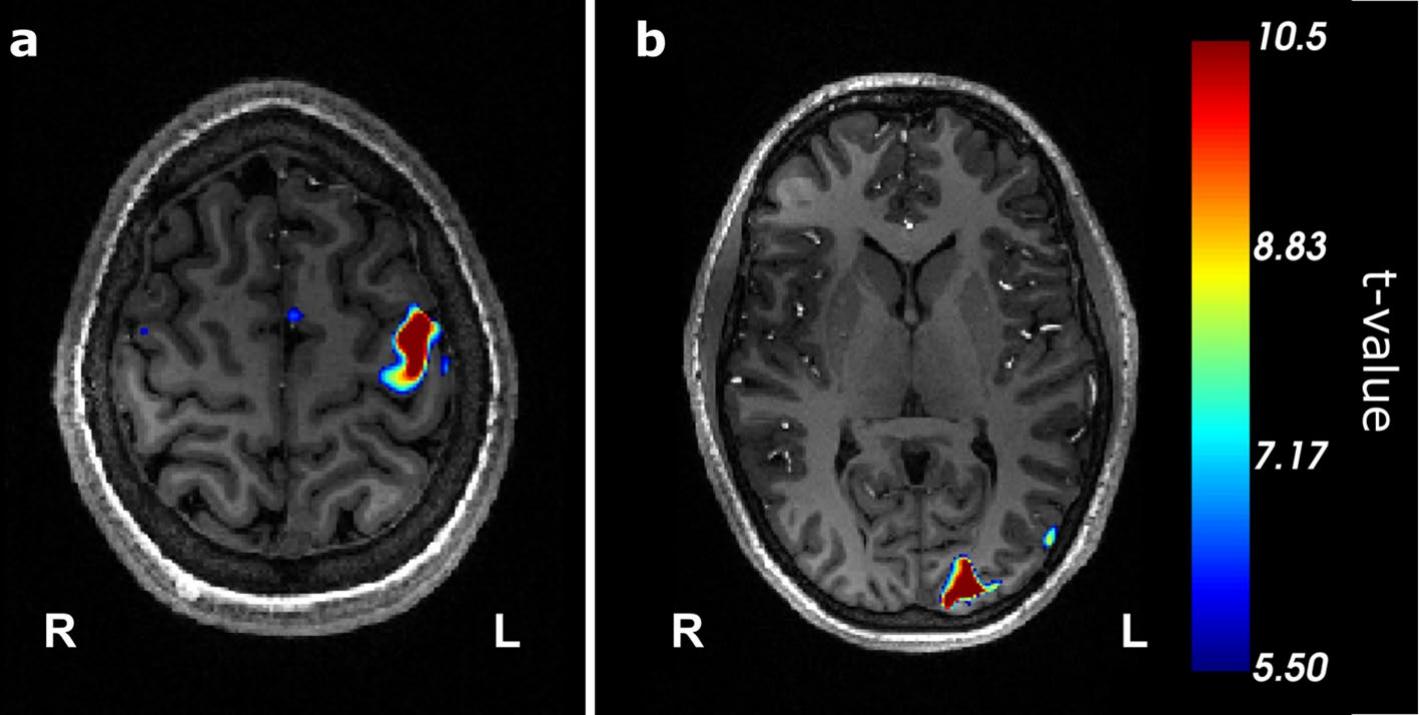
Representative functional MRI data (participant P2) depicting a BOLD map overlaid onto an MPRAGE image showing statistically probable activation within the left sensorimotor (a) and left visual cortex (b) employing the same paradigm as used for the oxygen experiments
